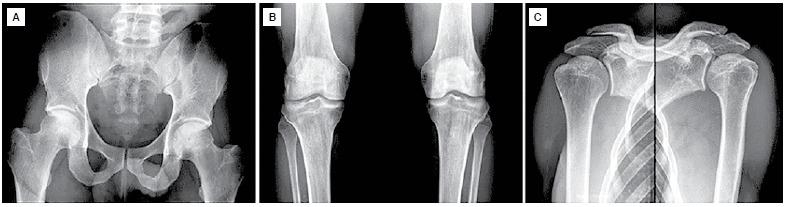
X-rays of hips (A), knees (B) and shoulders (C) showing preserved joint congruity.
